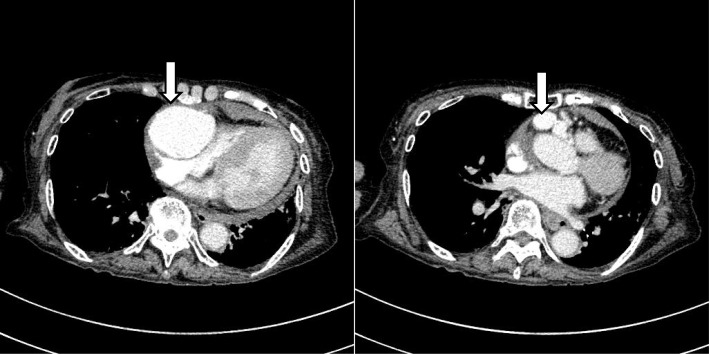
Initial computed tomography of the chest. Computed tomography of the chest shows multiple aneurysmal masses (arrows) in front of the heart communicating proximally with the right coronary artery
